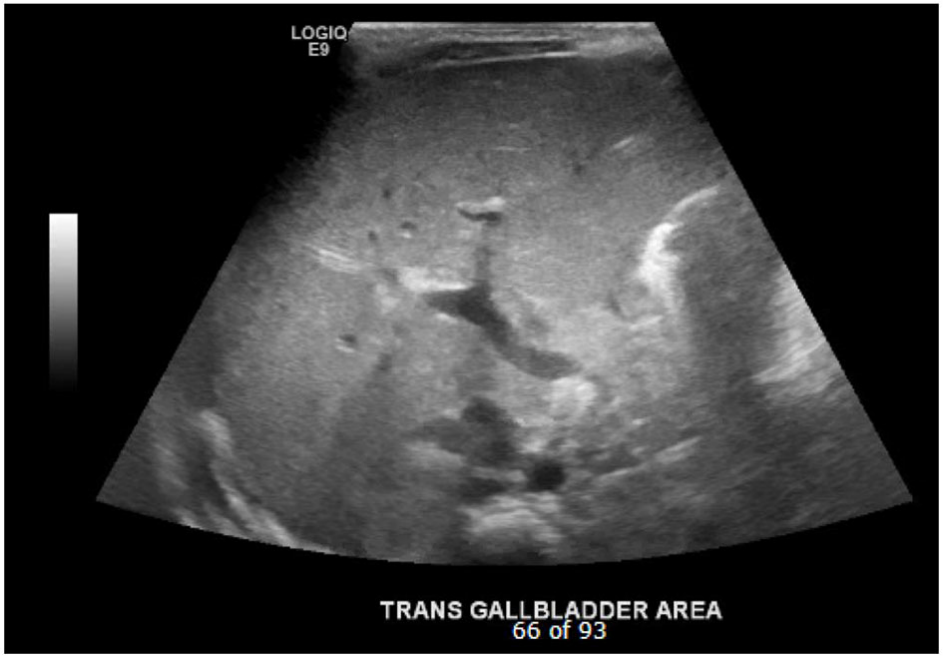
Absent gallbladder on ultrasound.
